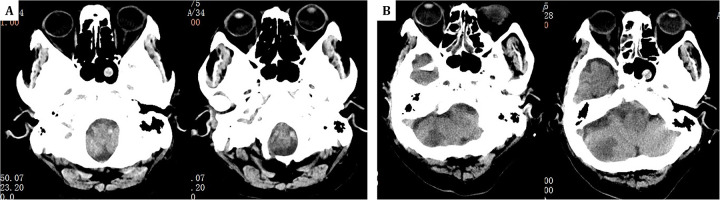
(A) One year prior, head CT revealed only a sphenoid sinus cyst. (B) The current admission's follow-up head CT at the same level now shows the sphenoid sinus cyst along with additional findings of right-sided ethmoid sinusitis.
